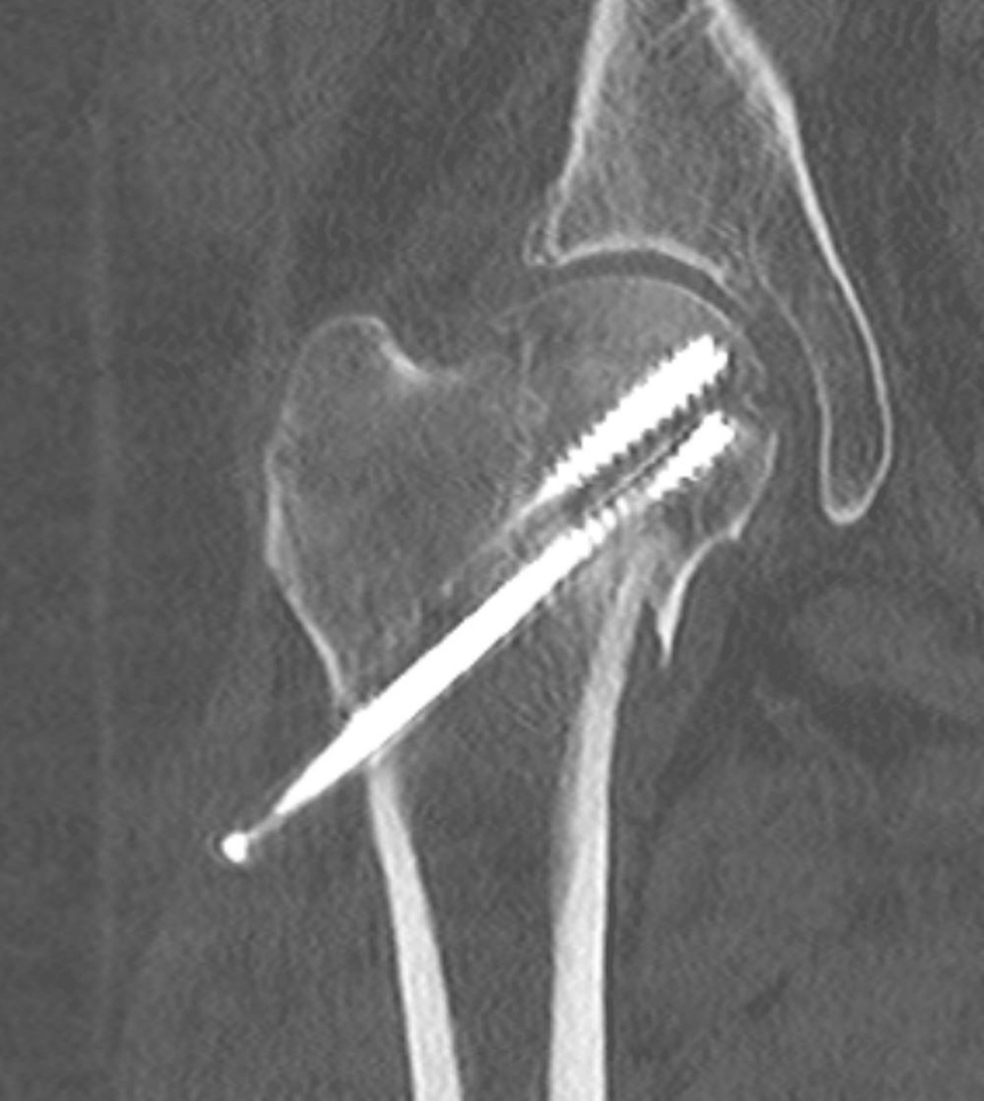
CT scan confirmed non-union at the fracture site
